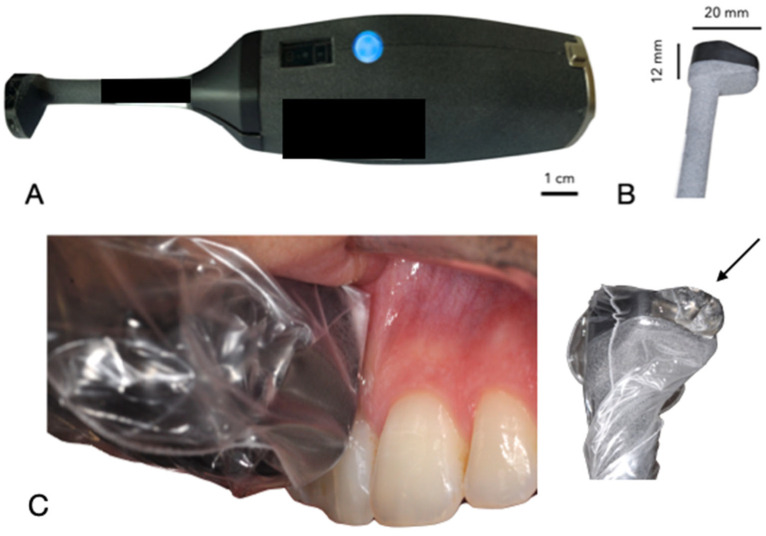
Intraoral ultrasound probe. (A) Probe design with ergonomic handle and (B) transducer head. (C) Intraoral positioning parallel to the tooth’s long axis, associated with a specialized intraoral coupling gel (arrow).
